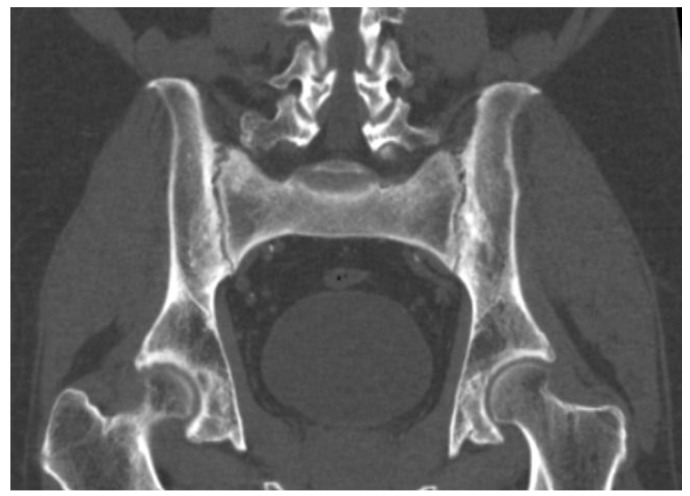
CT showing arthritic changes in the sacroiliac joints.
